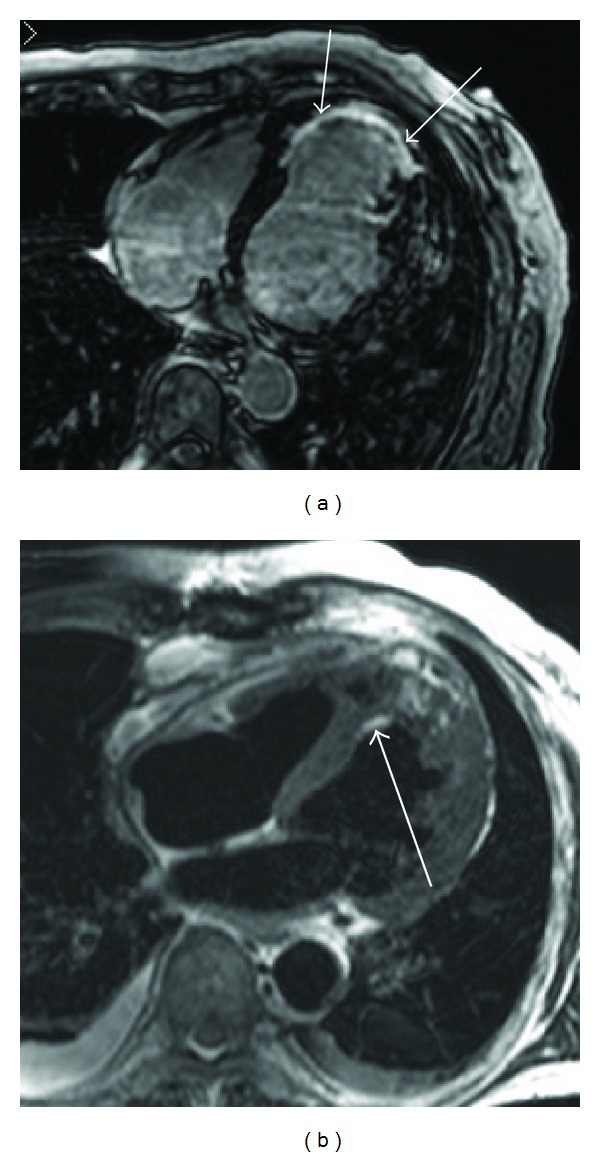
66-year-old male who has undergone cryoablation for ventricular tachycardia associated with chronic myocardial infarction. Delayed-enhancement MRI (a) visualizes apical myocardial scarring with various transmurality (arrows). T2-weighted MRI (b) shows myocardial edema after ablation (arrow), where nontransmural scarring has been seen in the delayed-enhancement MRI.
